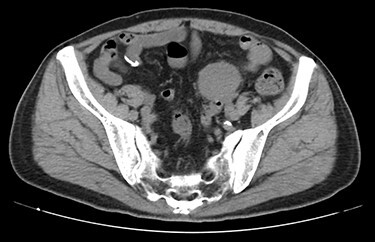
Contrast-enhanced CT showed a well-defined mass in contact with the small intestine that had heterogeneous enhancement.
